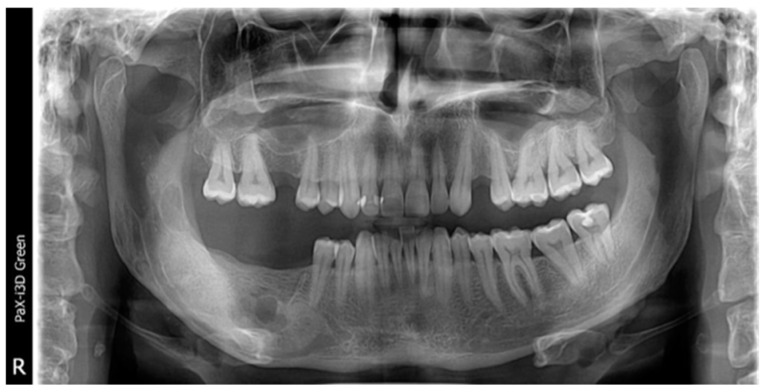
Orthopantomography, a year from recurrence surgery, revealed a bone healing process.
